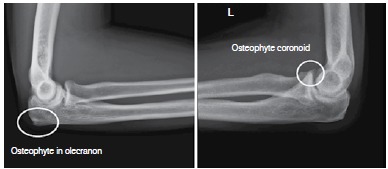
Radiographic alteration grade 2. Presence of osteophyte in olecranon and coronoid, shown in profile X-ray.
